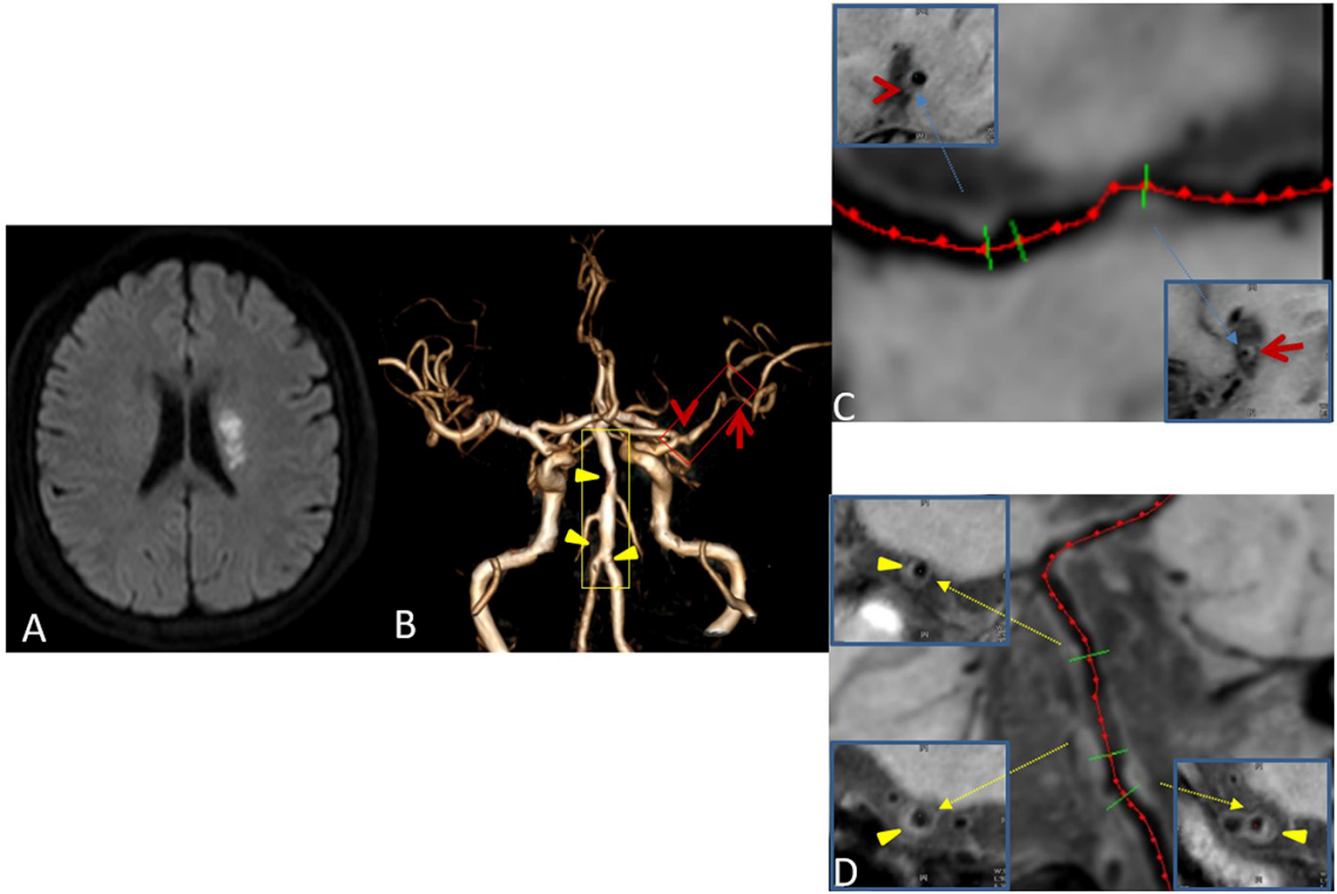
Plaque Classification. Male, 42 years old, presenting with sudden right limb weakness for 8 h. Diffusion-weighted imaging (DWI) shows acute left lateral paraventricular infarction (A). Time-of-flight magnetic resonance angiography (TOF MRA) shows stenosis at two sites in the M1 segment of the left middle cerebral artery (red box) and three sites in the basilar and left vertebral arteries (yellow box) (B). Curved planar reconstruction images show severe stenosis at the distal end of M1 as the culprit plaque (red arrow), and mild stenosis at the proximal end of M1 as an uncertain plaque (red Arrowhead) (C). The three plaques of the basilar artery and left vertebral artery are non-culprit plaques (Yellow triangular arrowheads) (D).
